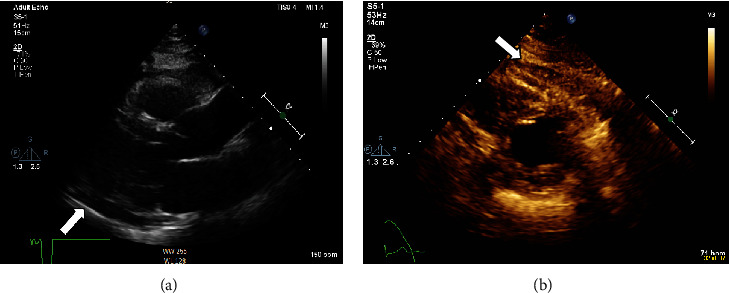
Transthoracic echocardiogram in the long parasternal axis view (a) and short axis view (b).
